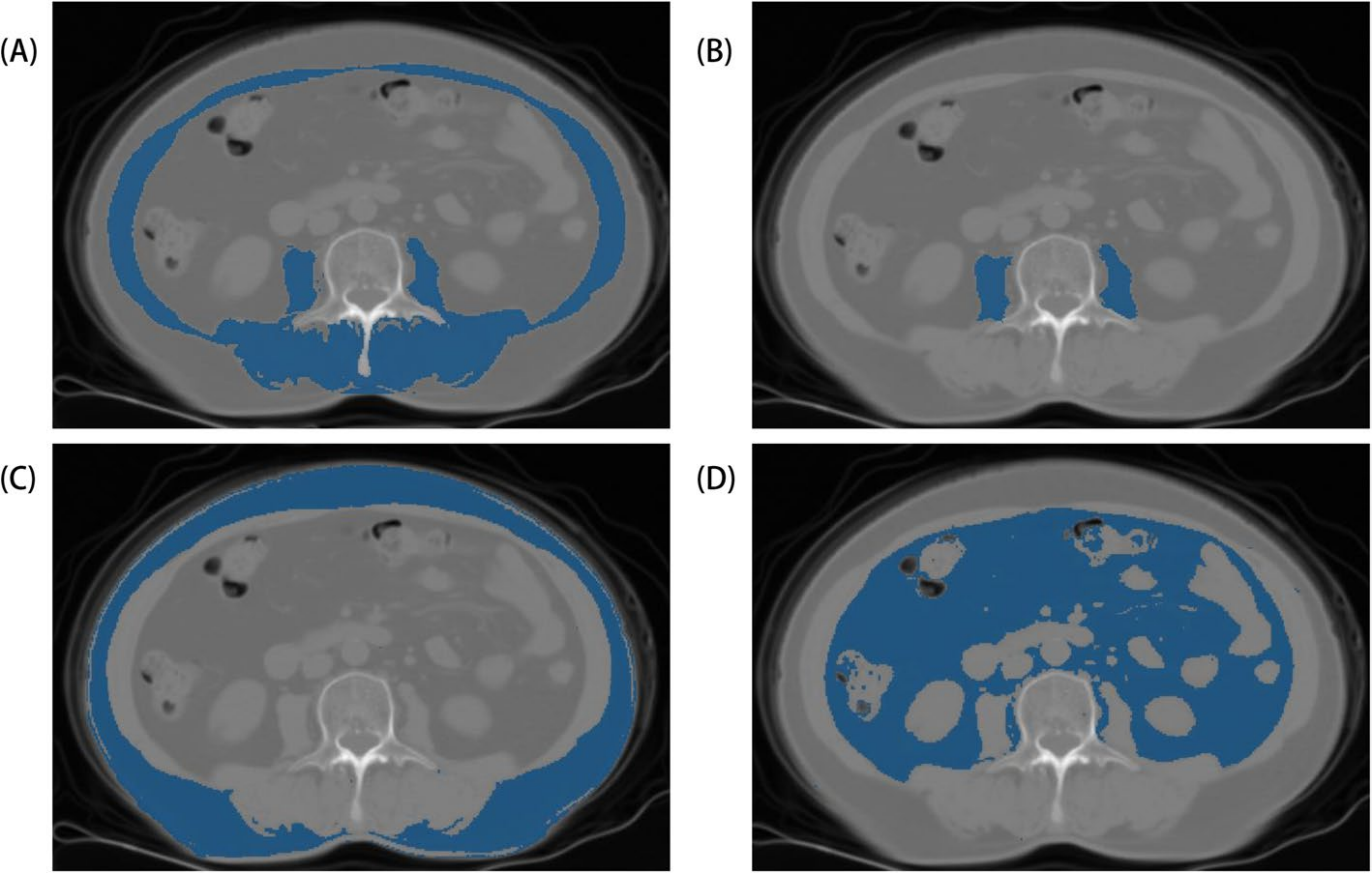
Measurement of the skeletal muscle index (A), psoas muscle index (B), subcutaneous fat index (C) and visceral fat index (D) using computed tomography at the L3 level
